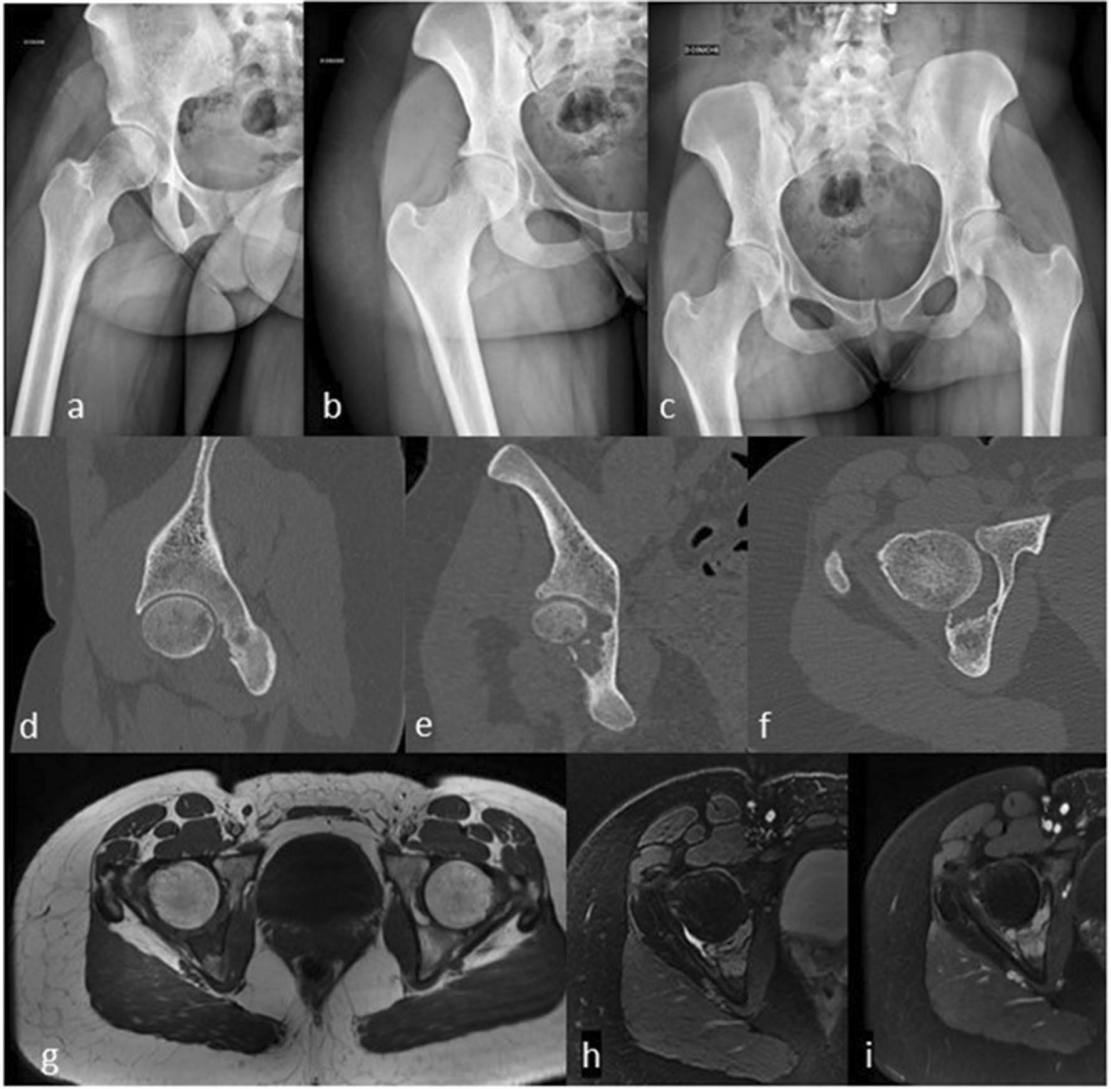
Case of Langerhans cell histiocytosis. A 24 years-old female, no smoker and no drinker has been experiencing left hip pain in the last 6 months relieved by Cortisone injection, with recurrence of the pain 4 months later. No general status loss was observed. There was an inflammatory syndrome on blood test. On palpation, pain was localized on the ischium and on the mobilization of the hip without sensitive or motor dysfunction. (1a/c) Standard radiographs of the hip. (1d/f) Sagittal, frontal, and axial views on computed tomography. (1g/i) T1 and T2 axial and T1gado axial views on magnetic resonance imaging.
